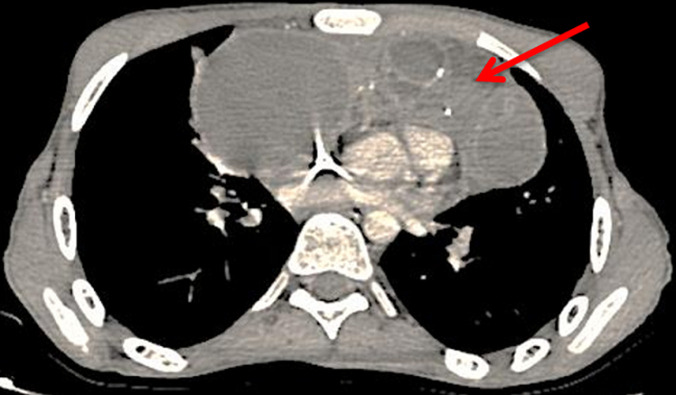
chest computed tomography scan revealing a mediastinal mass
